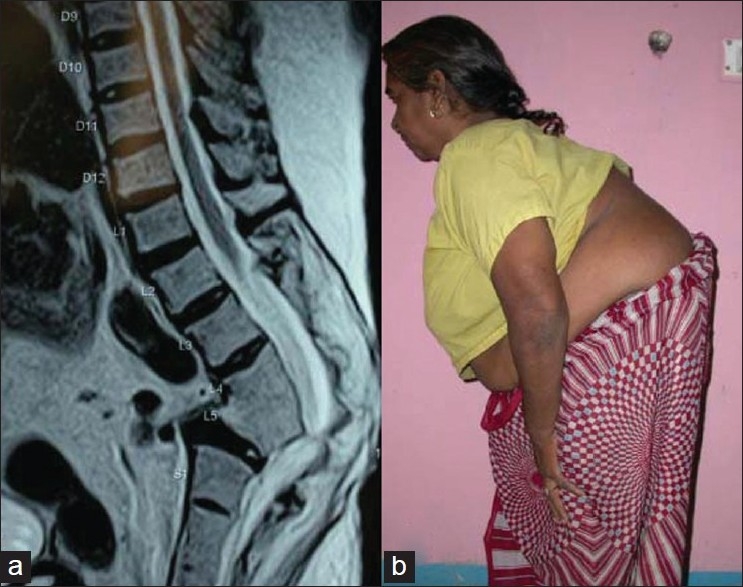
T_2_ weighted MRI of lumbosacral spine (a) shows a healed kyphosis with lumbar canal stenosis. Clinical photograph (b) of the same patient shows the posture with forward bending due to severe neurogenic claudication
